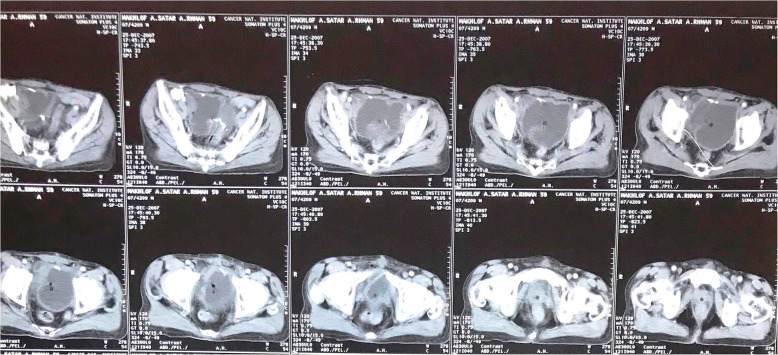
2-year postoperative CT shows post-evacuation cuts of IC neobladder after PRC. Pouch has 485 ml capacity. Anterior and posterior staple remnants are present without stones or encrustations on them
